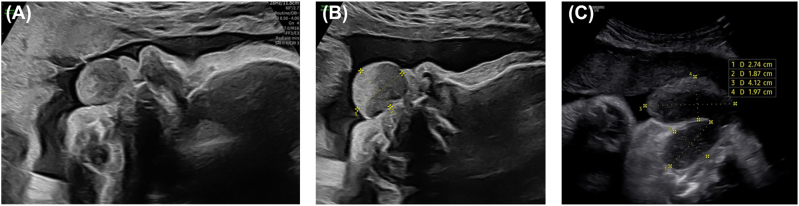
Prenatal ultrasound images: (A) masses (epulis) that surge through the fetus’s mouth at 32 weeks and 4 days of gestation; (B) progressively enlarging epulis at 33 weeks and 6 days of gestation; (C) two components of the epulis at 37 weeks and 0 days of gestation.
